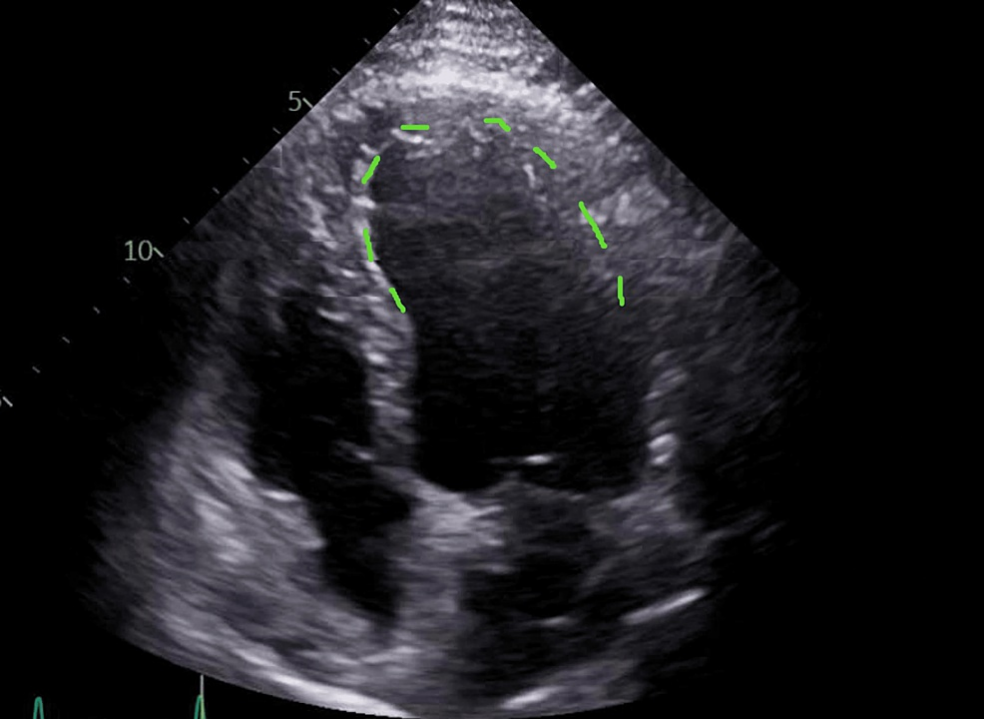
Echocardiographic findings suggestive of TCM with characteristic apical ballooning (shown with green dotted line). TCM, Takotsubo cardiomyopathy
